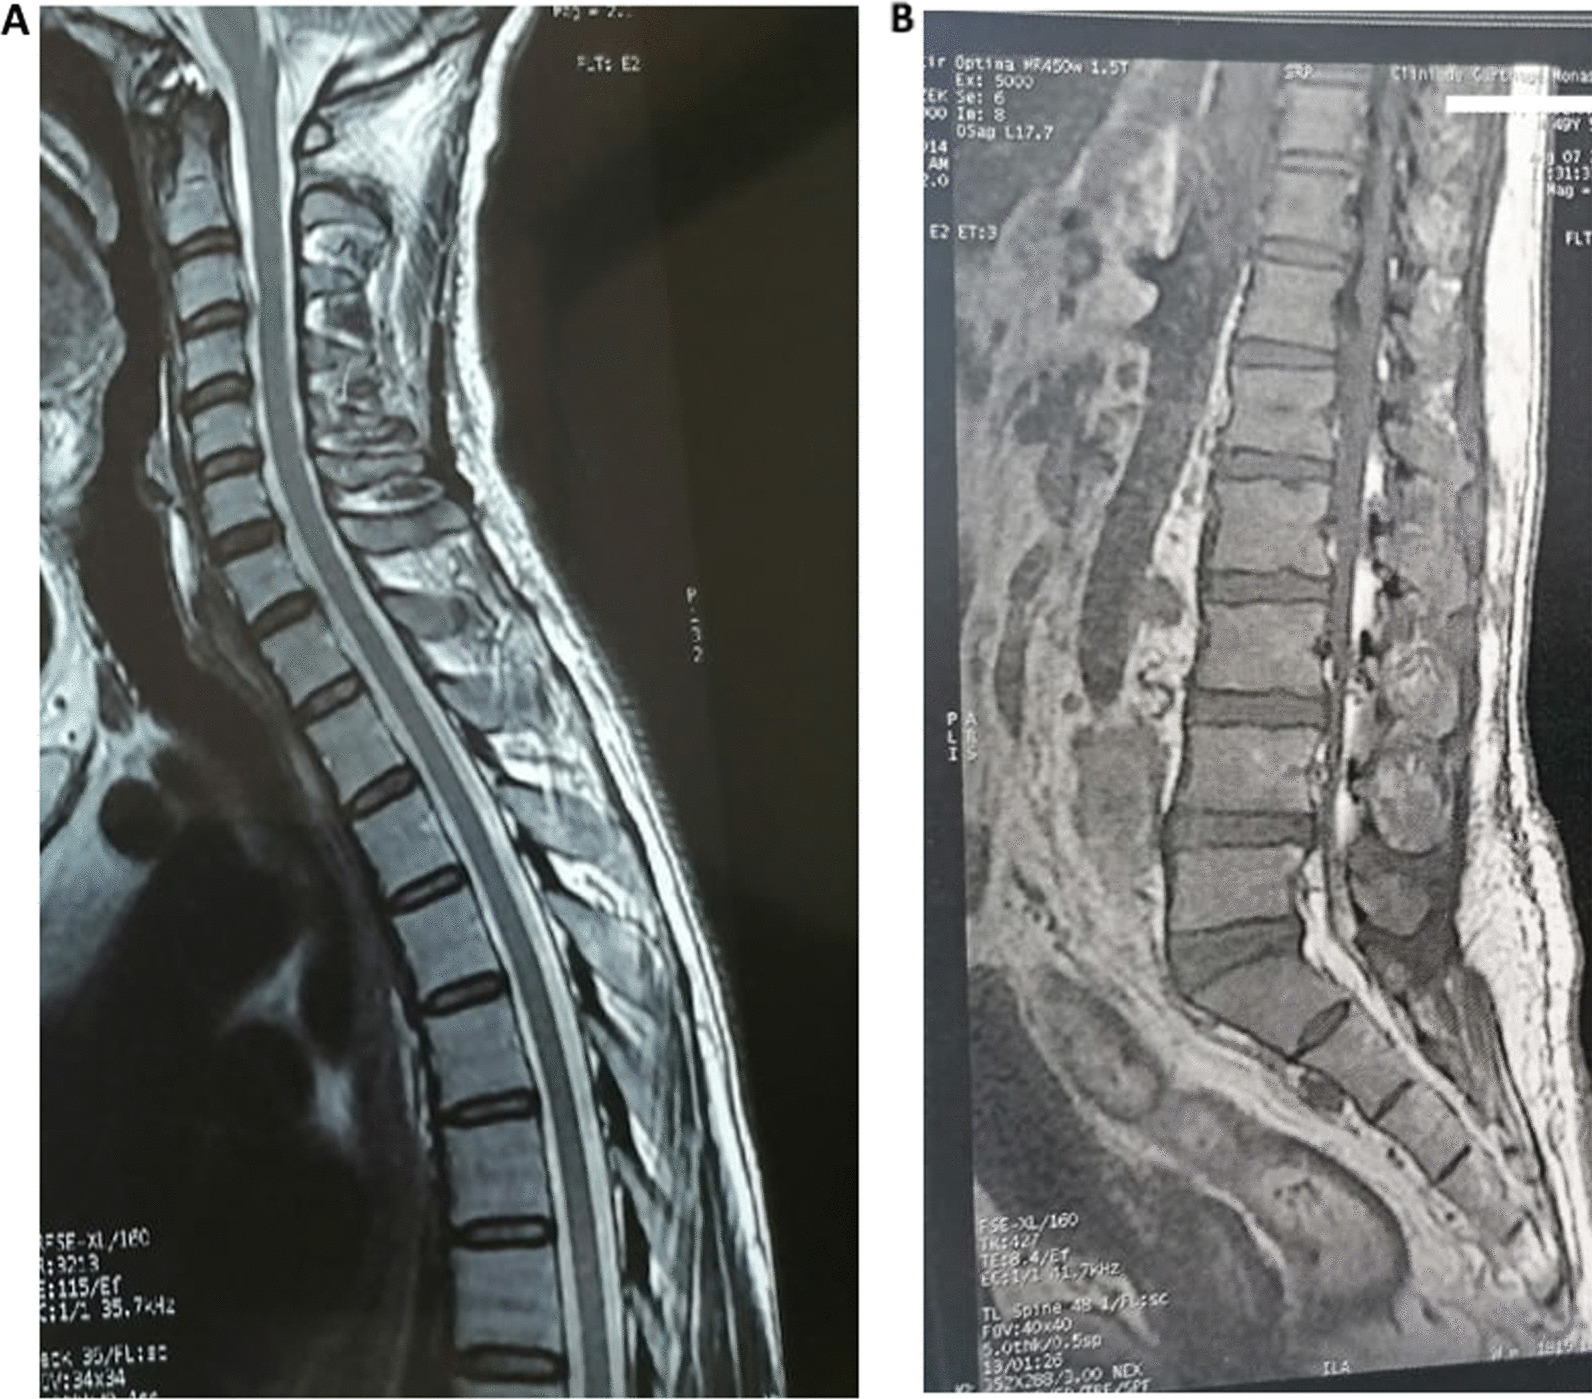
Spinal cord magnetic resonance imaging (A T1 with Gado, B T2): No abnormalities
